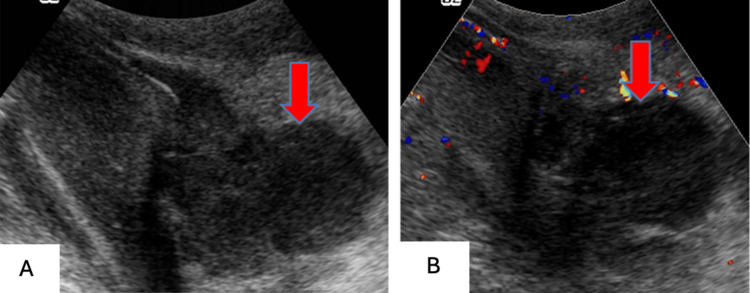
An anechoic and avascular pooling within the scrotal wall shown on grayscale (13A) and color Doppler (13B) US images (red arrows).
